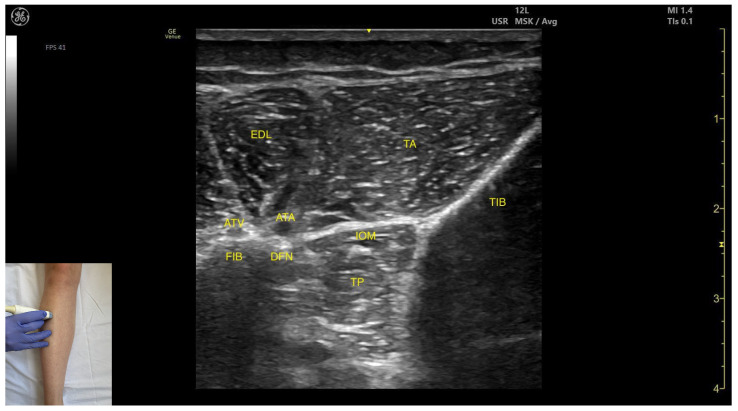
Ultrasound anatomy of the tibialis posterior—anterior window: ATV—anterior tibial vein; ATA—anterior tibial artery; FIB—fibula; DFN—deep fibular nerve; EDL—extensor digitorum longus; TA—tibialis anterior; IOM—interosseous membrane; TP—tibialis posterior; TIB—tibia.
